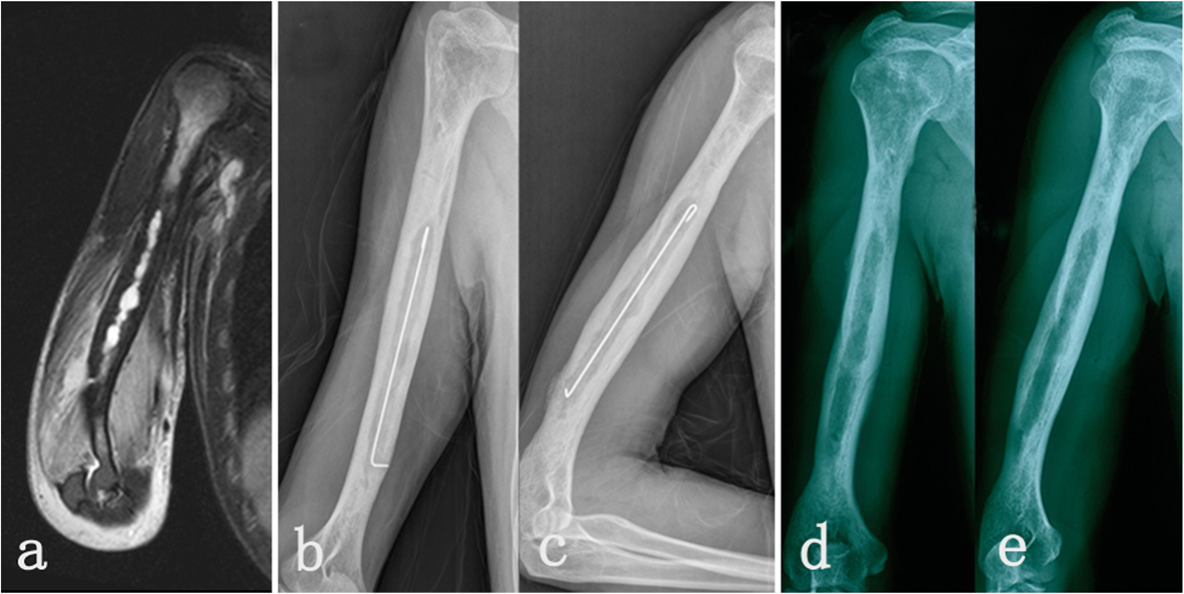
Imaging results from a 23-year-old male with type I humeral osteomyelitis (Patient 4). a Preoperative magnetic resonance imaging of the medullary infection in the humerus. b-c Positive and lateral X-ray after debridement showing medullary management with an antibiotic-coated cement rod. d-e Positive and lateral X-ray after removing the cement rod in the second stage
